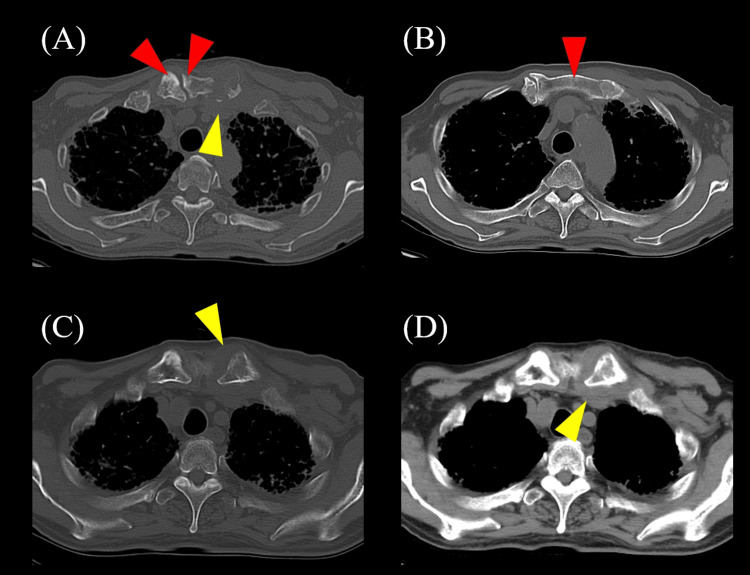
Results of the chest computed tomography (CT) five months after stroke onset. (A, B, C) Chest CT showing joint capsule thickening, joint effusion (yellow arrowheads), and signal changes in the sternoclavicular joint and surrounding bone: sternum and clavicle (red arrowheads).
